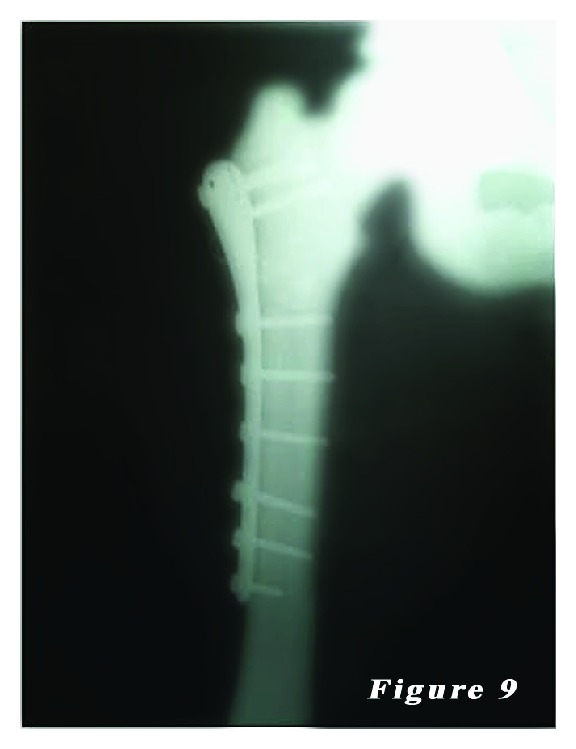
Her last right femur radiography. It looks like fracture union.
